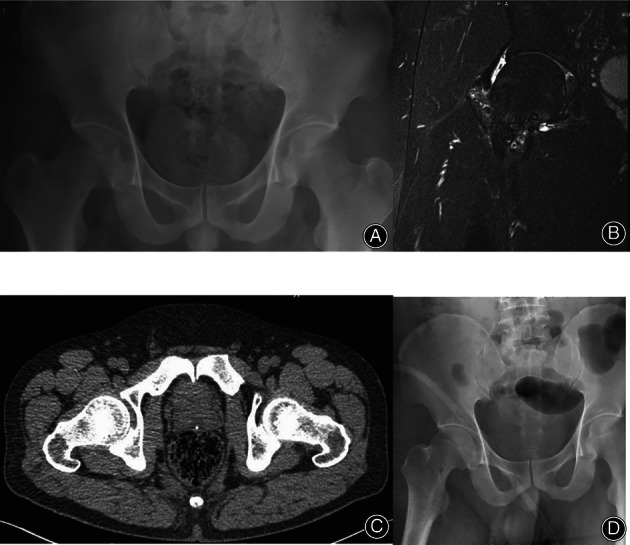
The images of hip PVNS received arthroscopy. (A) Preoperative pelvic radiograph depicting the right hip to PVNS. (B) Preoperative MRI. (C) Preoperative CT 3D reconstruction of hip PVNS. (D) Postoperative pelvic radiograph
